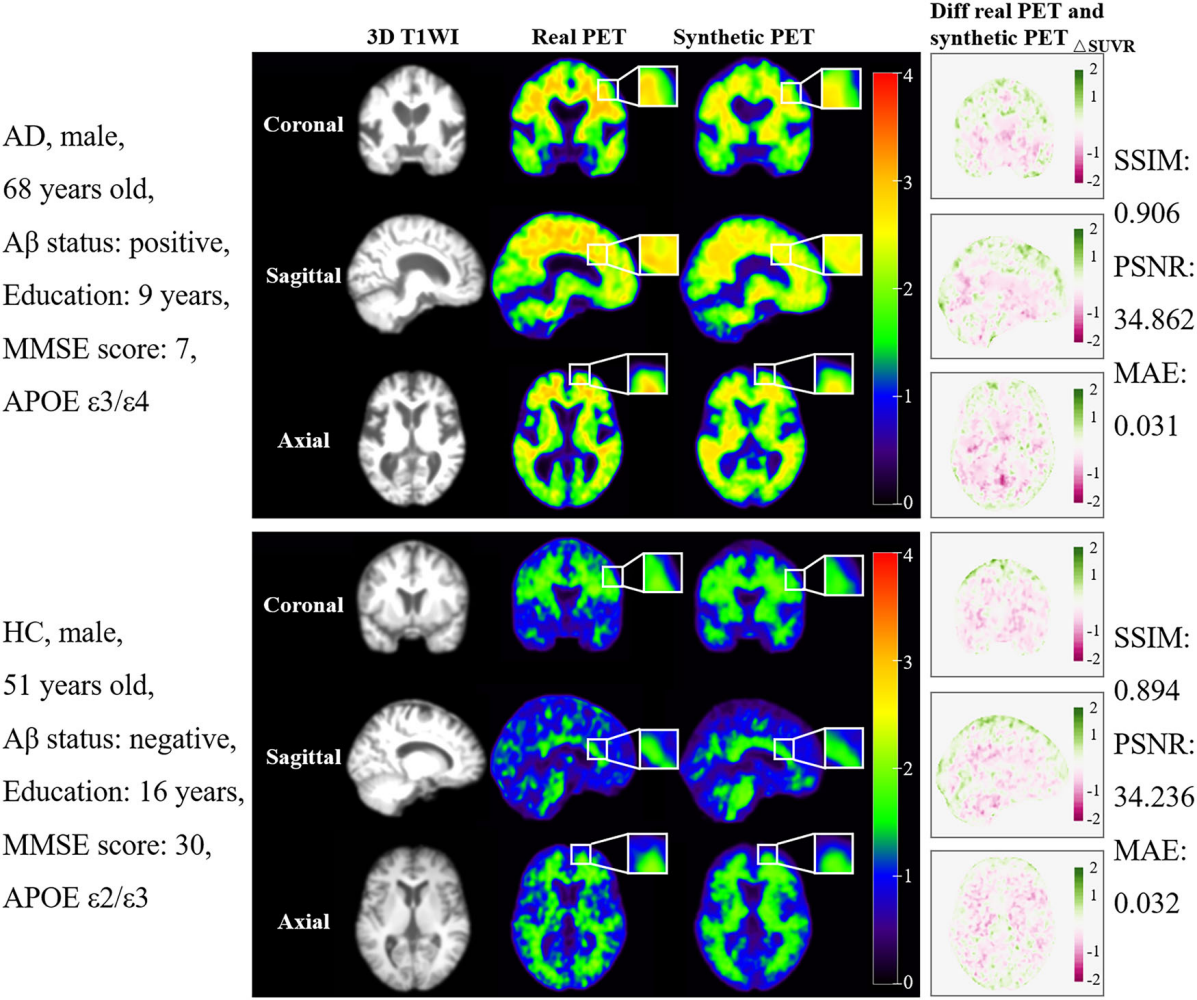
Representative model generated synthetic PET images for two subjects. In the panel 4 columns can be seen: the MRI input image (left), the real PET image (center-left), the synthetic PET image (center-right), and the error map between real and synthetic PET (right). The color bar of real and synthetic PET represents SUVR concentrations, and the error map color bar is the difference in SUVR. AD, Alzheimer’s disease; Aβ, amyloid-β; HC, healthy controls; MMSE, Mini-Mental State Examination; APOE, apolipoprotein E; 3D T1WI, high-resolution T1-weighted imaging; PET, positron emission tomography; diff, difference; △SUVR, the difference in standardized uptake value ratio; SSIM, structural similarity index measure; PSNR, peak signal-to-noise ratio; MAE, mean absolute error
